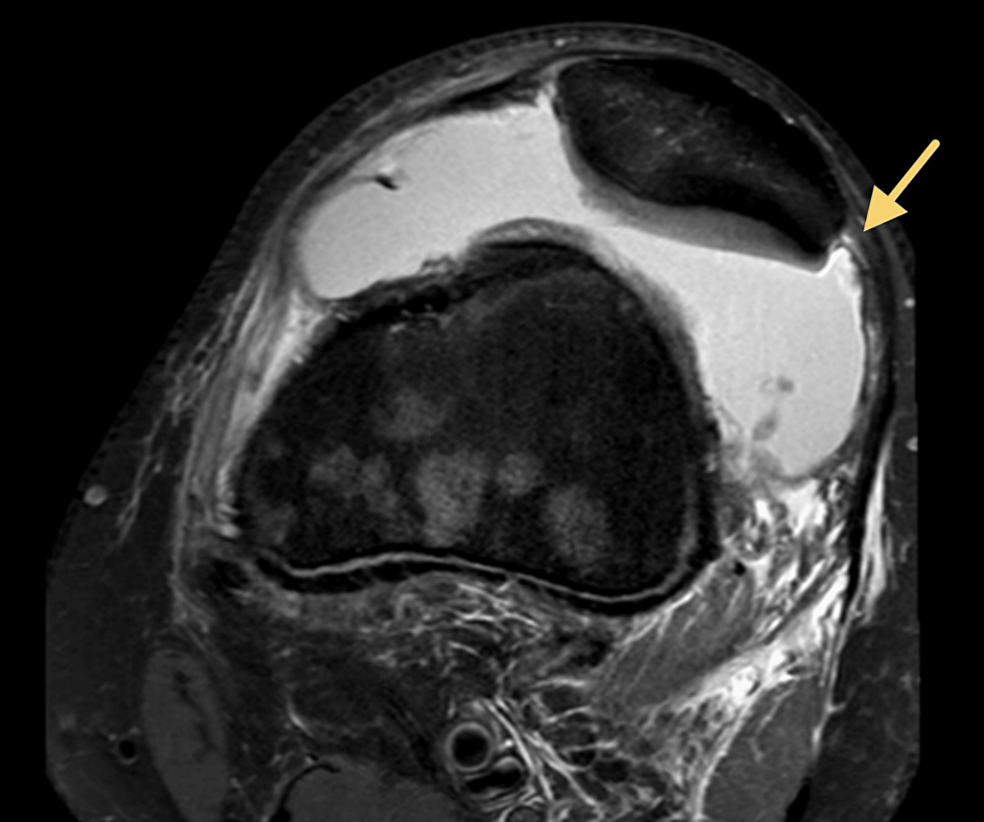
MRI of the knee of the third patient, demonstrating severe effusion, trochlear dysplasia, and severe lack of patellar containment. The arrow indicates the region of severe effusion, trochlear dysplasia, and the severe lack of patellar containment.
